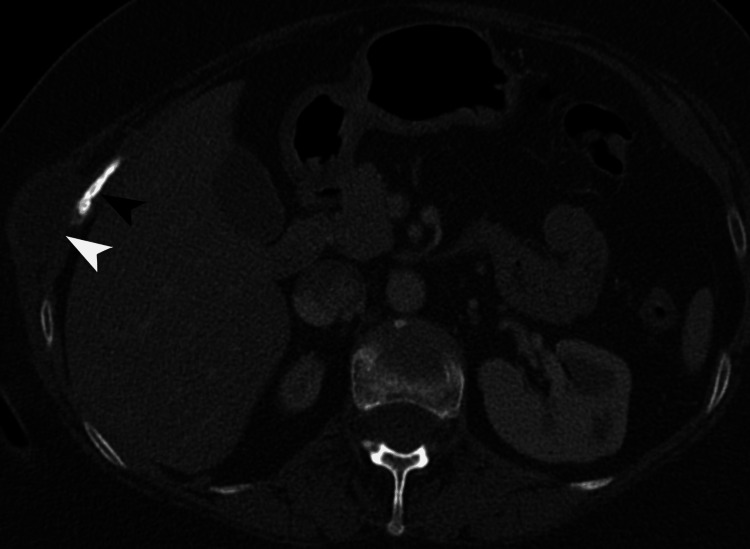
Right chest wall cystic lesion with rib erosion. Axial contrast-enhanced CT of the abdomen, in the bone window, demonstrates a well-defined cystic lesion (white arrowhead) with peripheral enhancement, measuring 3.3 × 2.4 cm, in the right chest wall, causing erosion of the ninth rib anterolaterally (black arrowhead). CT: computed tomography
